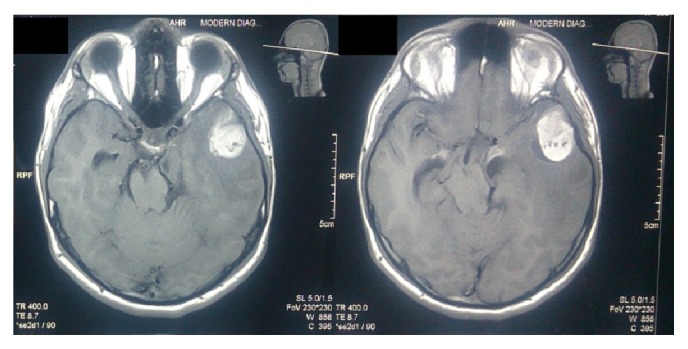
Preop MRI showing the lesion in the left temporal lobe with no dural attachment.
